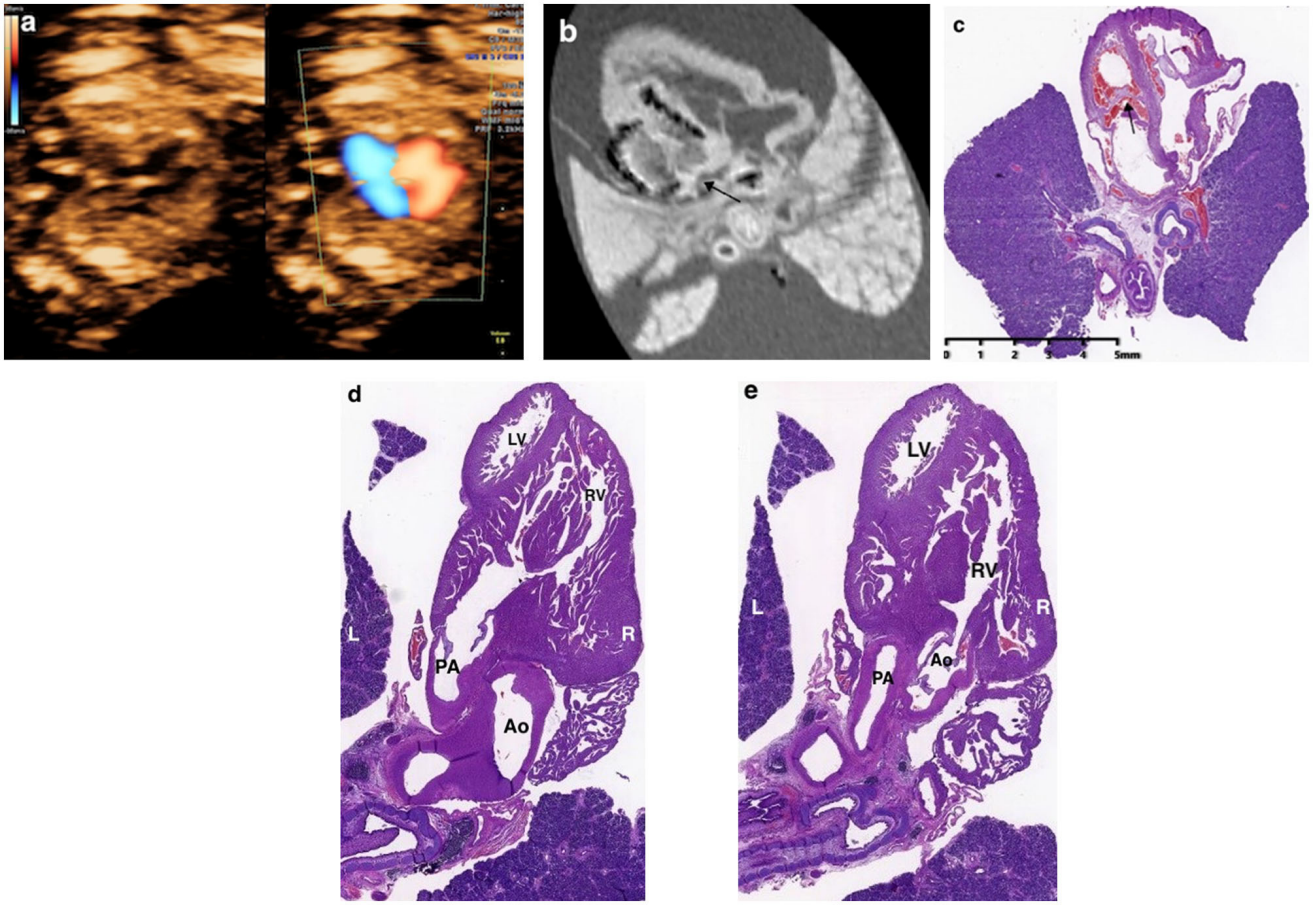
Discordant findings of postmortem MRI and autopsy compared with prenatal ultrasound. A fetus (Case 17 in Table 2) was detected as transposition of the great arteries (a) and was terminated at 17+6 weeks of gestation for Trisomy 18. The postmortem MRI showed both aortic artery and pulmonary artery arose entirely from the right ventricle with the aorta to the right and anterior to the pulmonary artery. (b,c) The autopsy confirmed the diagnosis of double-outlet right ventricle (d,e).
